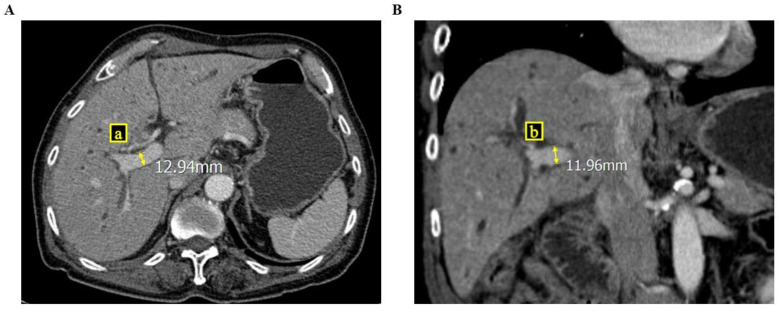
These figures show how to measure the cross-sectional area of the right portal vein via computed tomography ((A) cross section view; (B) coronary section view), where a and b are the semi-major and minor axes of the right portal vein.
